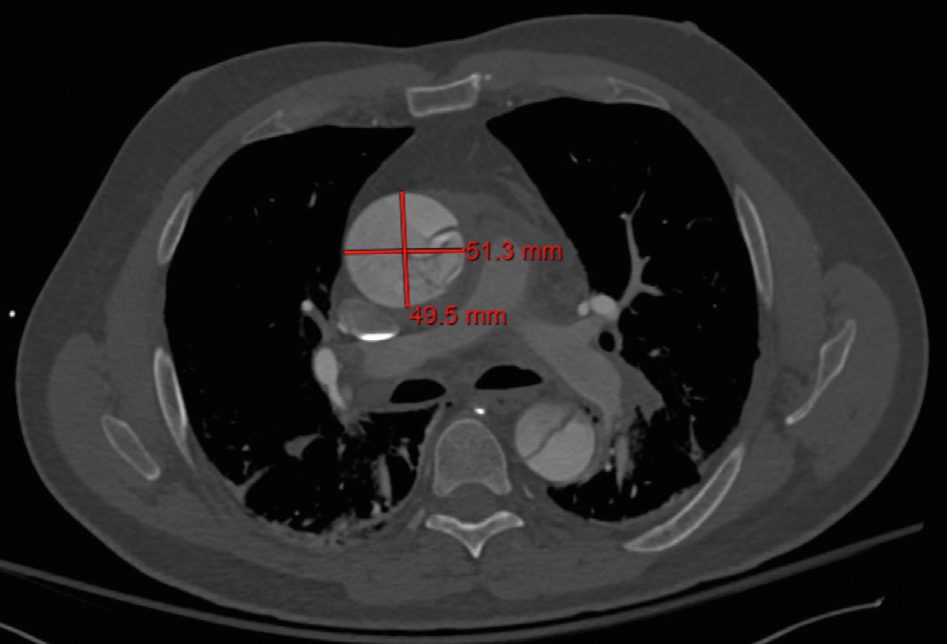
Computed tomography image with measurement of total aortic diameter.
